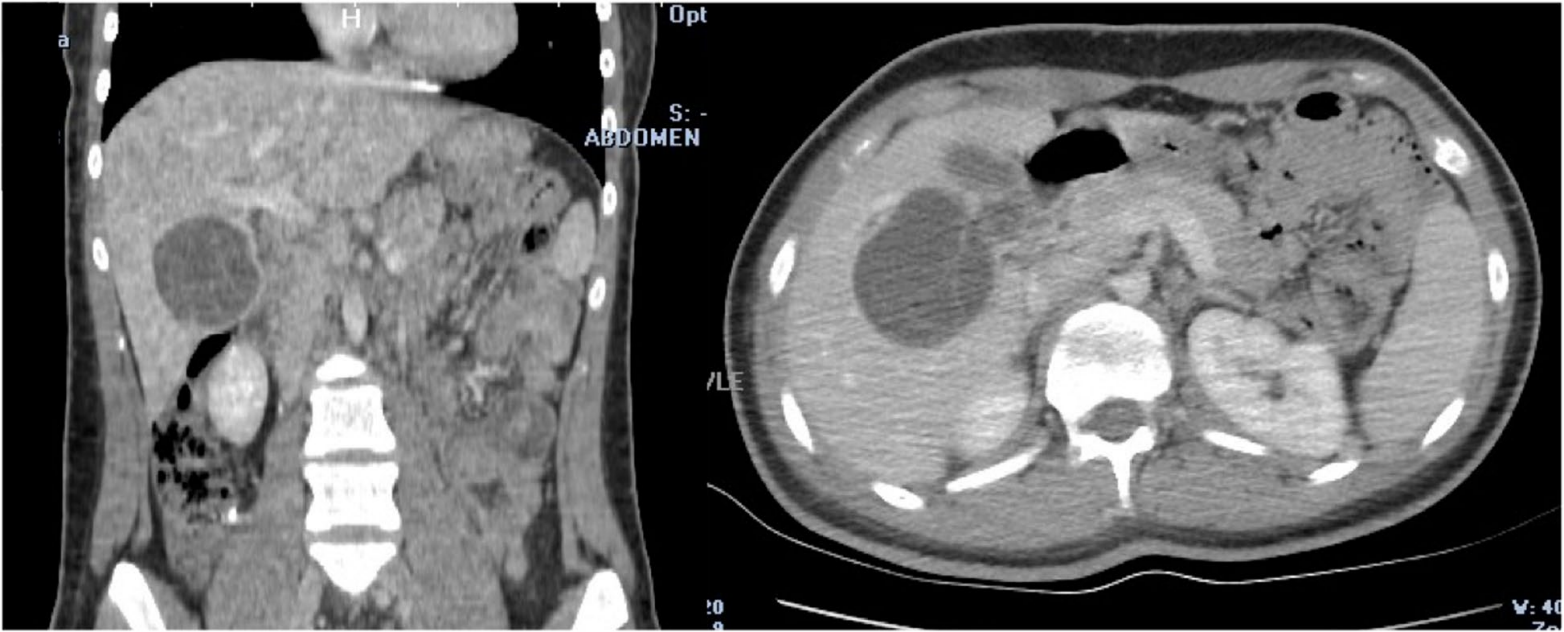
Abdominal computed tomography images. Image shows ruptured hepatic hydatid cyst, inside of which floating membranes are present
